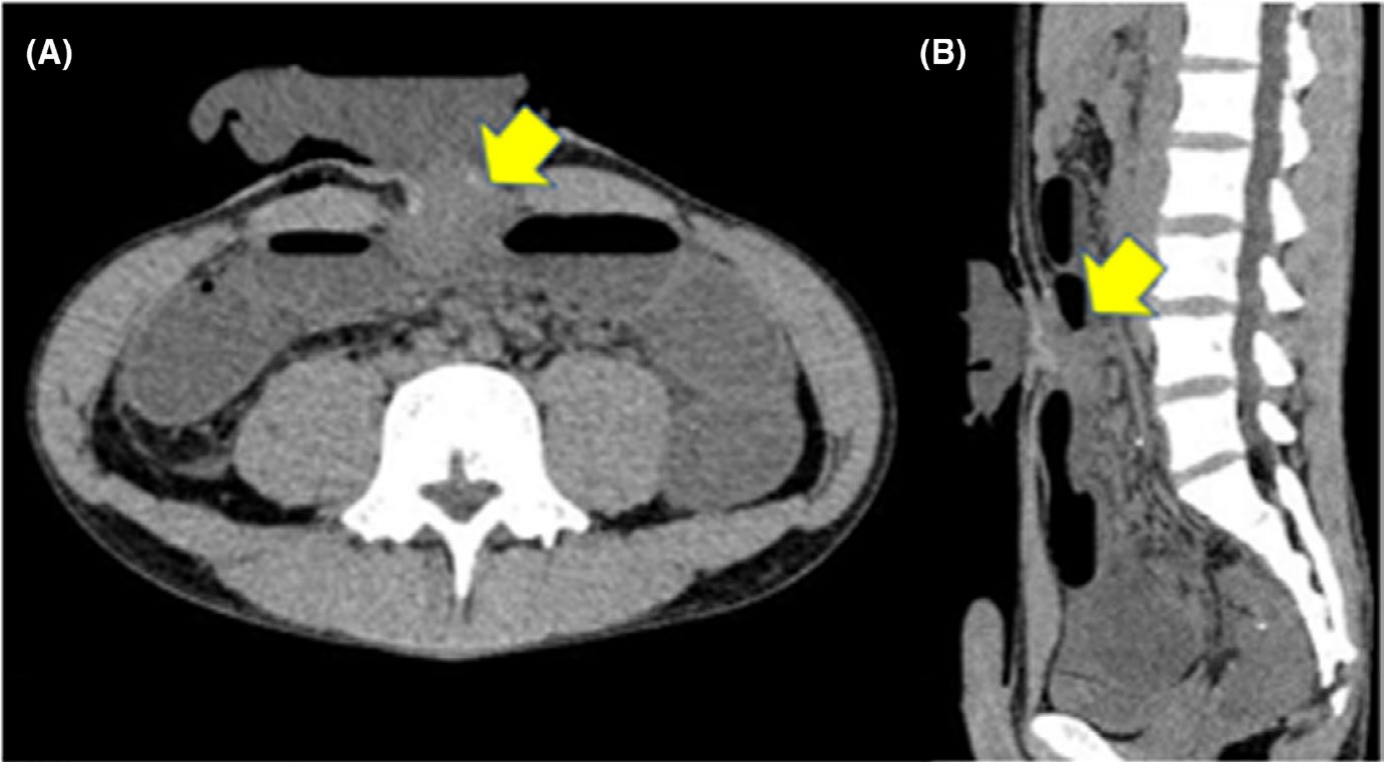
Representative CT images of stoma outlet obstruction (SOO). The arrow points to the area where the intestine was obstructed at the site of a diverting ileostomy. a: Axial image. b: Sagittal image
